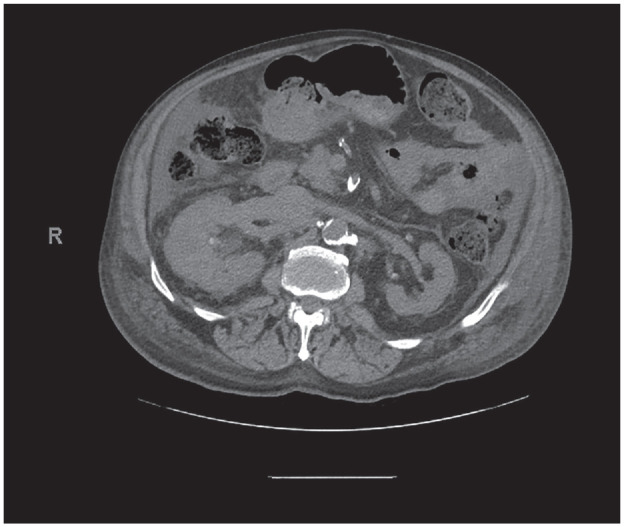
A noncontrast CT scan of the abdomen revealed asymmetric fullness of the right kidney with hydroureteronephrosis, with an increased density concerning for soft tissue neoplasm in the right ureter. There was a concern by the interpreting radiologist for an infiltrative renal mass (arrow).
